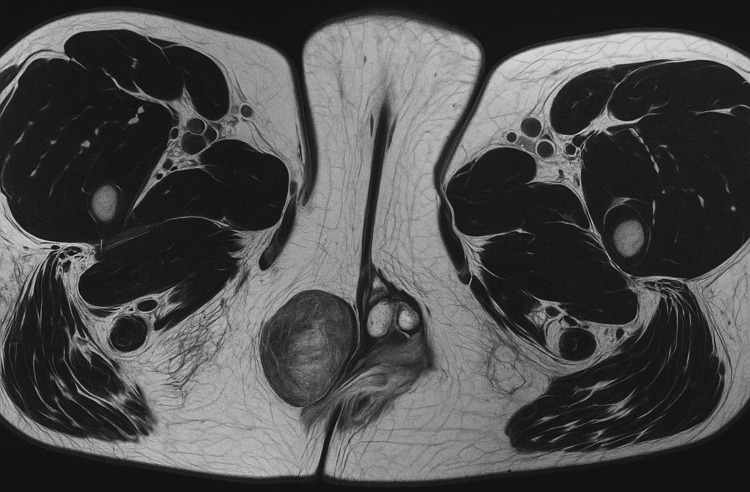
Postoperative MRI of the perineum, demonstrating multifocal confluent soft tissue nodules consistent with vulval leiomyoma.
